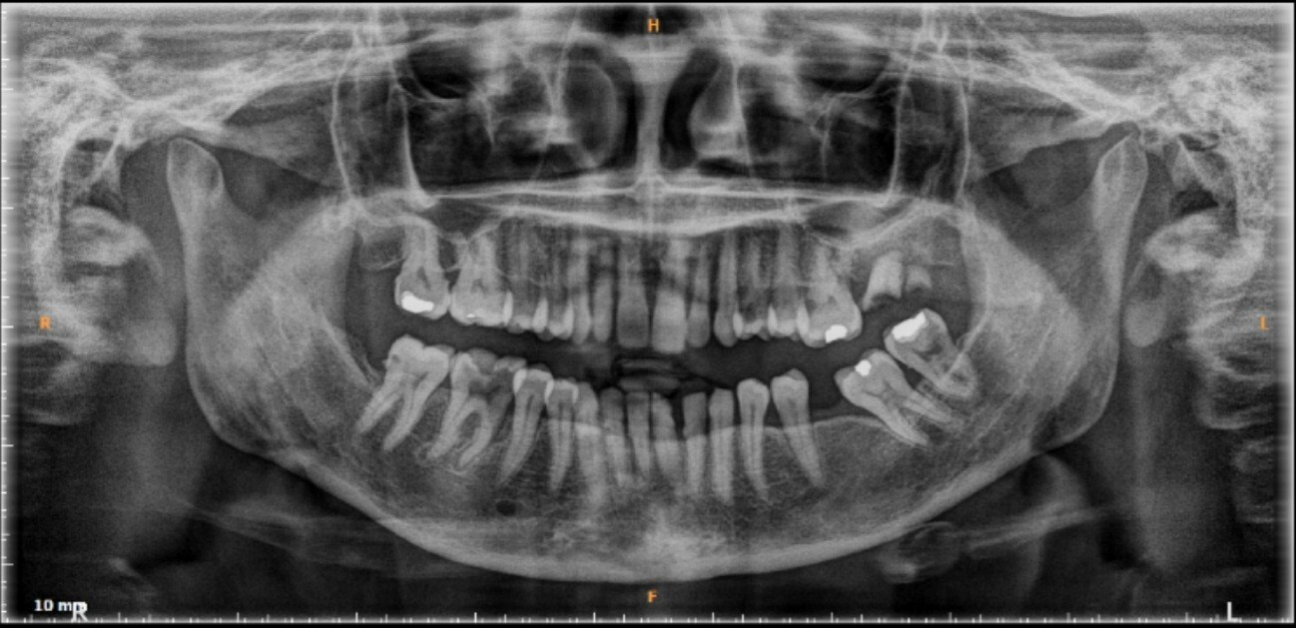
Panoramic X-ray revealed multiple missing teeth, teeth radix, tooth extrusion, and tooth with periapical lucency.
